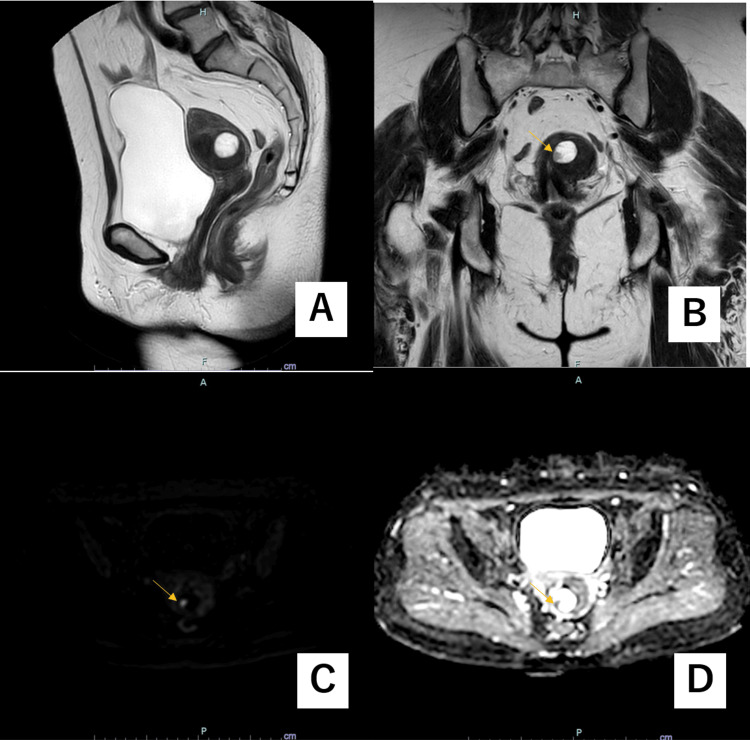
Pelvic contrast-enhanced magnetic resonance imaging The image shows a 39-mm mass in the uterine body, predominantly having a low intensity on T2-weighted images, suggesting leiomyoma containing a cystic lesion with a small solid component (indicated by the arrow).  This solid component appeared to be high intensity on T2-weighted images (A, sagittal, and B, coronal), high intensity on diffusion-weighted images (C, transaxial), and low value on ADC map images (D, transaxial). ADC, apparent diffusion coefficient
